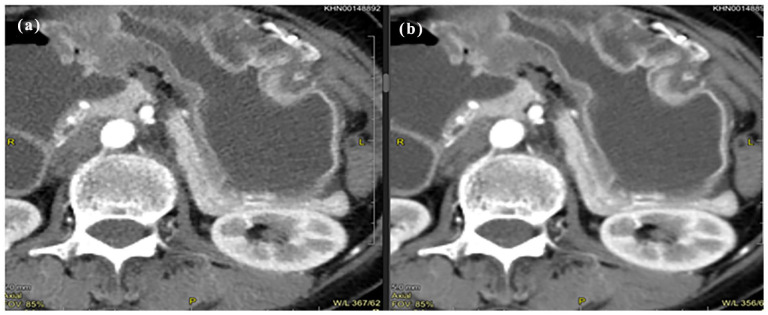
Idose4 (a) versus Precise Image (b) for arterial phase abdomen and pelvis CT.
